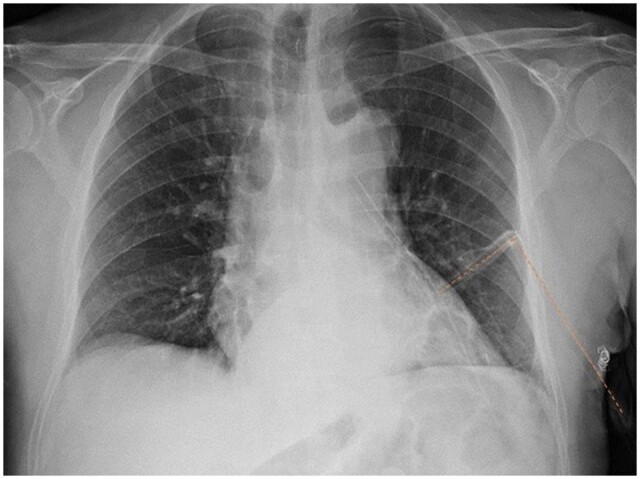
Example of T-tube kinking on chest X-ray (highlighted with dotted line).
